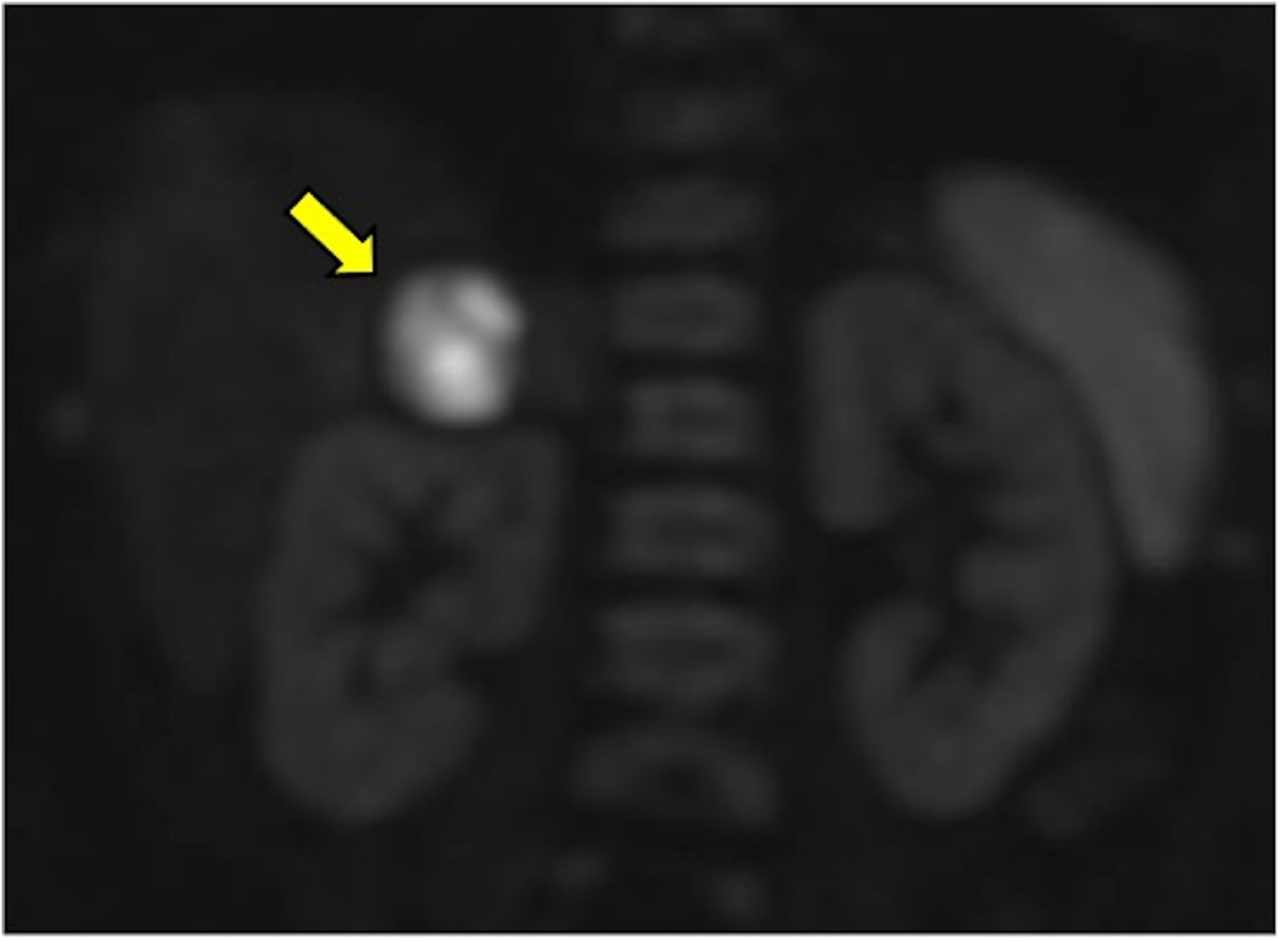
A 6-month-old child with fUTI; DW-MRI showed no focal renal areas of restricted diffusion. Right adrenal hemorrhage (arrow).
